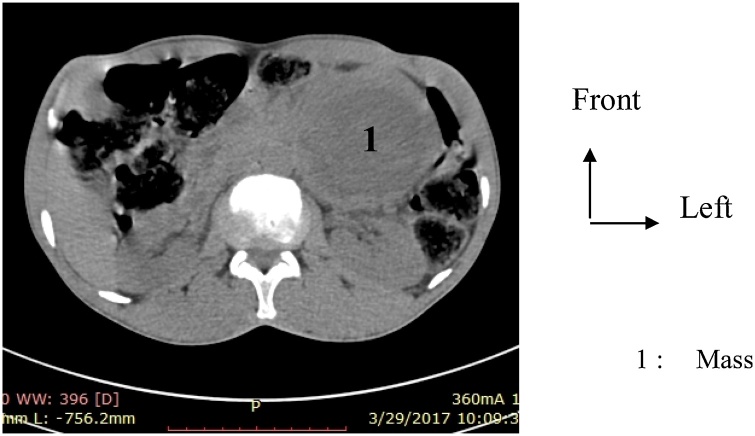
CT scan image mimicking a cancer of the tail of the pancreas.
